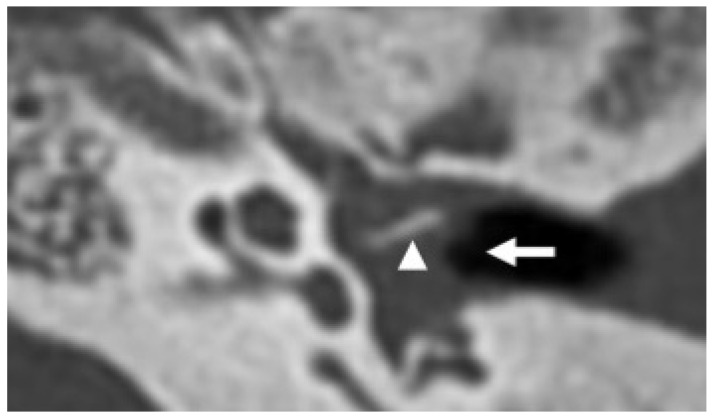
Varicella-zoster* virus encephalitis in a 20-year-old patient. Axial CT shows fluid in the left middle ear (arrow) due to chronic otitis. There is also marked erosion of the ossicular chain, with the long process of the incus being the only remaining appreciable ear bone left (arrowhead).
